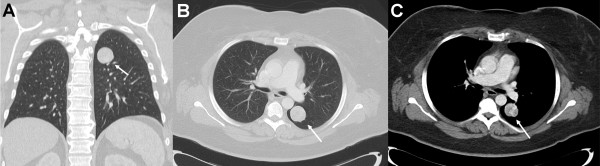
Computed tomography of the chest. (a) Coronal and (b, c) axial plane. The glomangioma is marked with an arrow.
